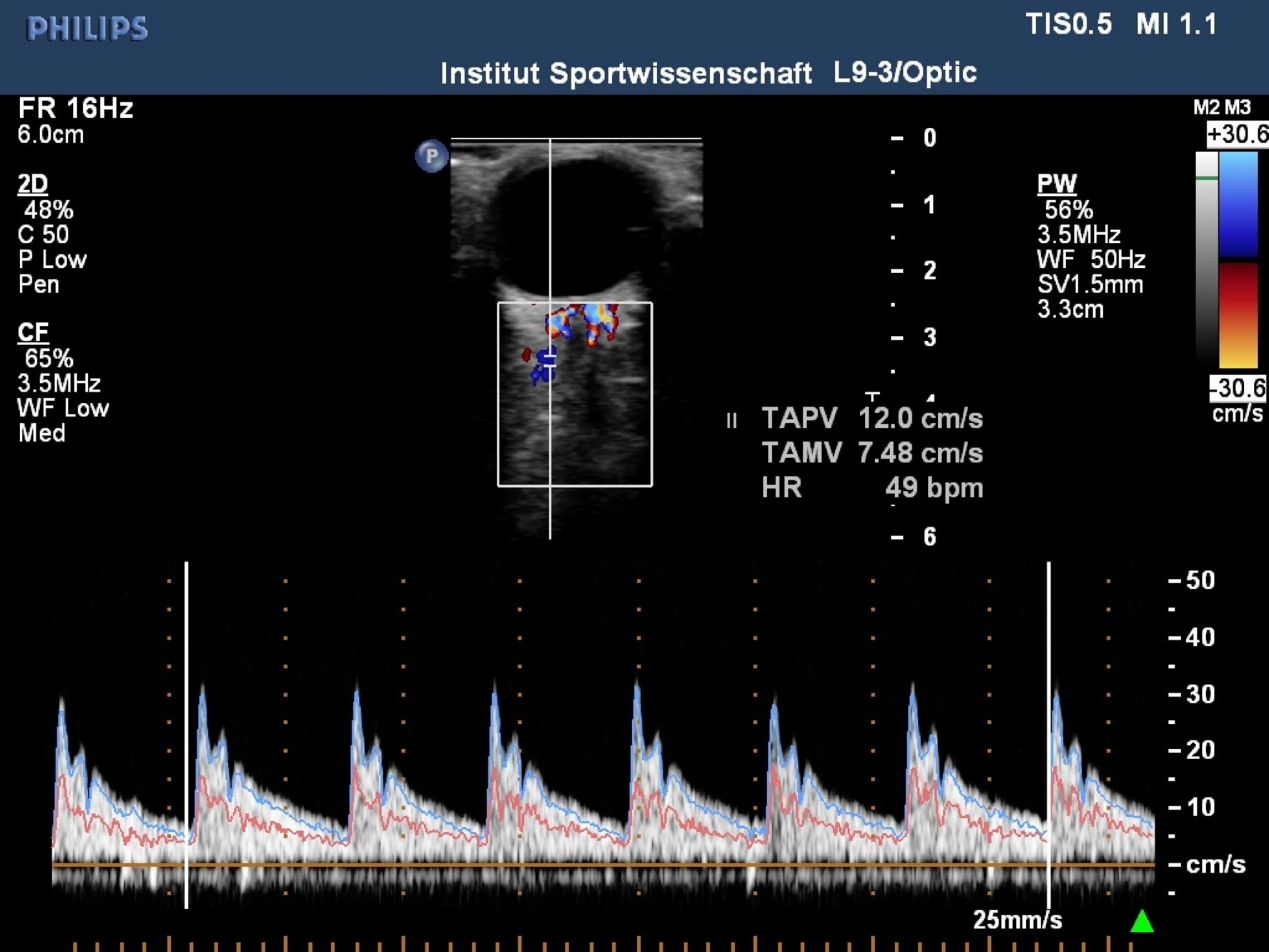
Example of PCAv ultrasound image and velocity trace
